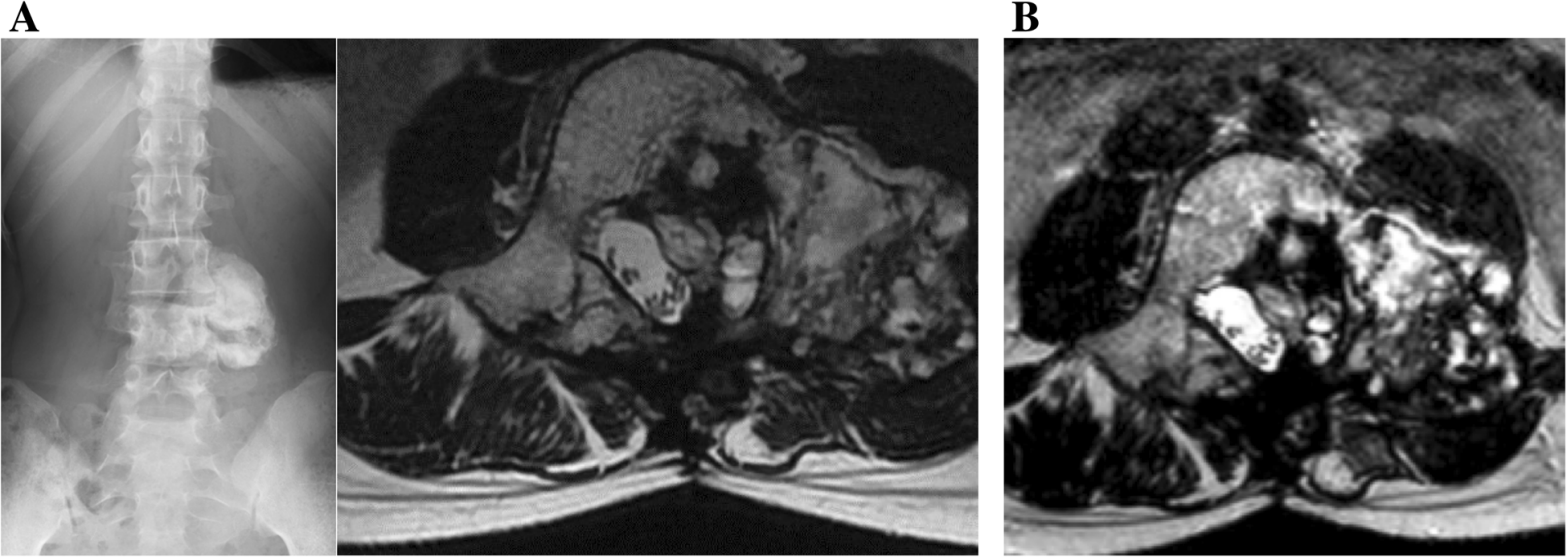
a X-ray and T2 axial MRI at 2 years after carbon ion radiotherapy. b The same level of T2 axial MRI 10 years later. No significant recurrence was observed
